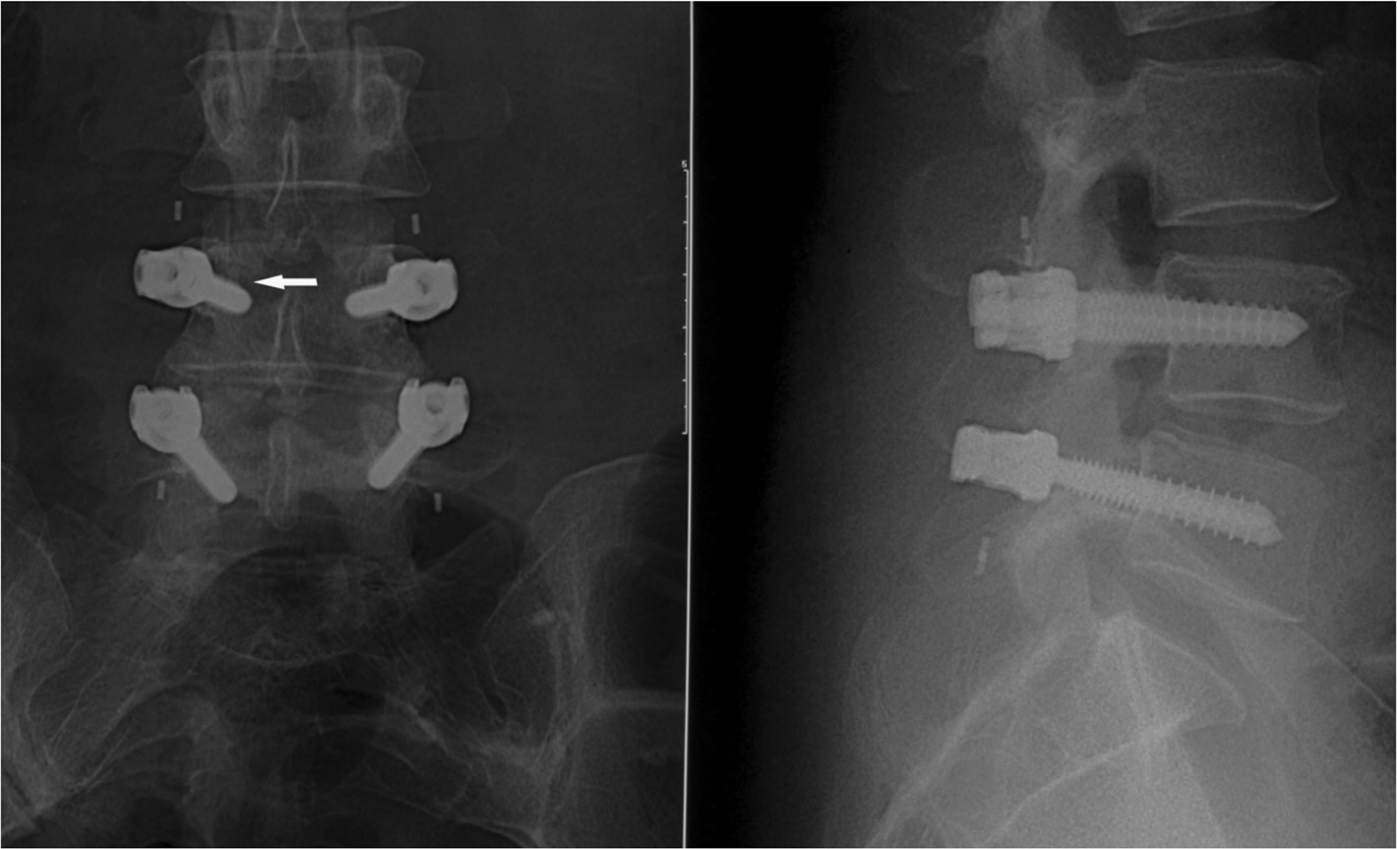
X-ray radiographs showed halo sign (*white arrow*) around right L4 pedicle screw, which indicated screw loosening
